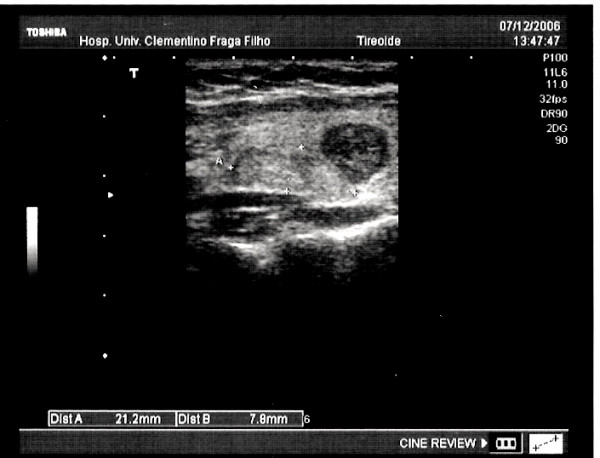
Ultrasonography of the thyroid gland with visualization of heterogenous nodule in the left lobe.
